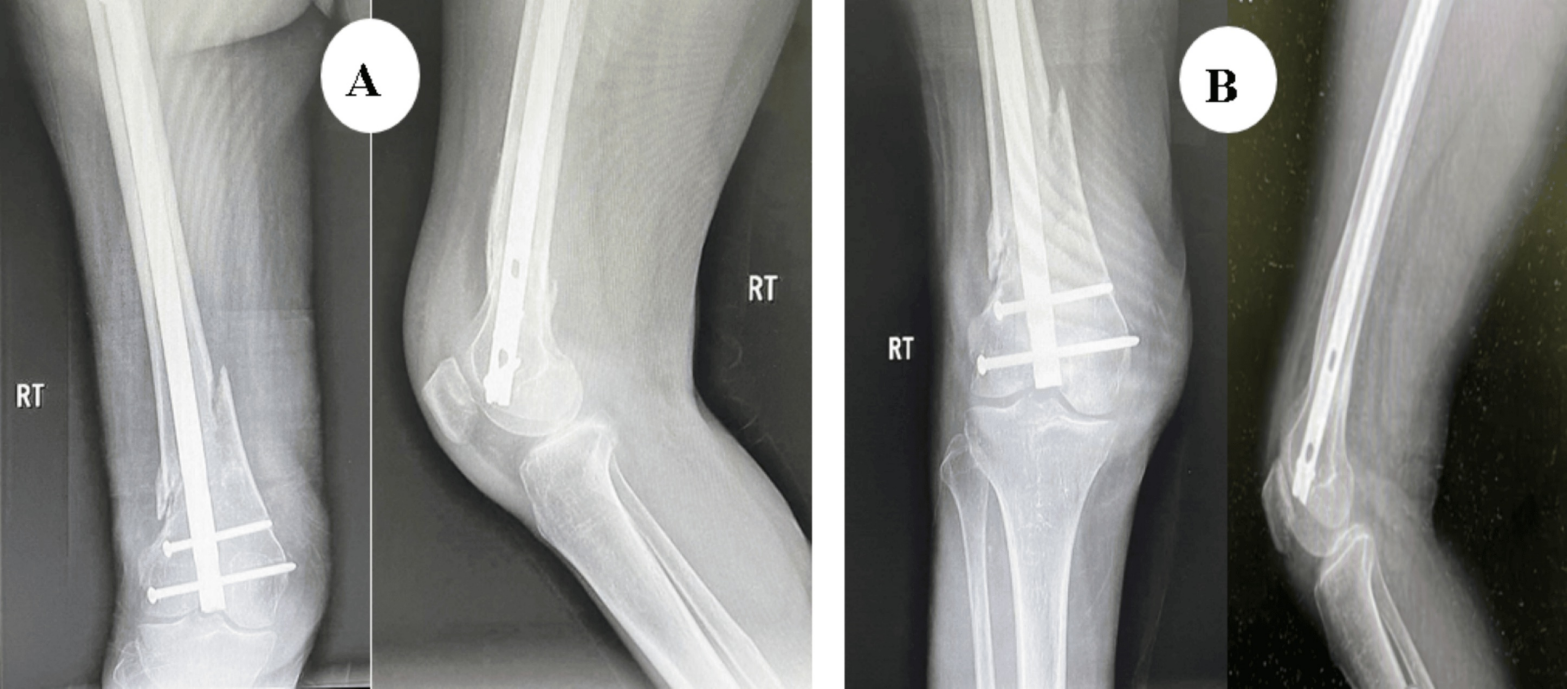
Case 6 follow-up X-rays at (A) three and (B) six months
